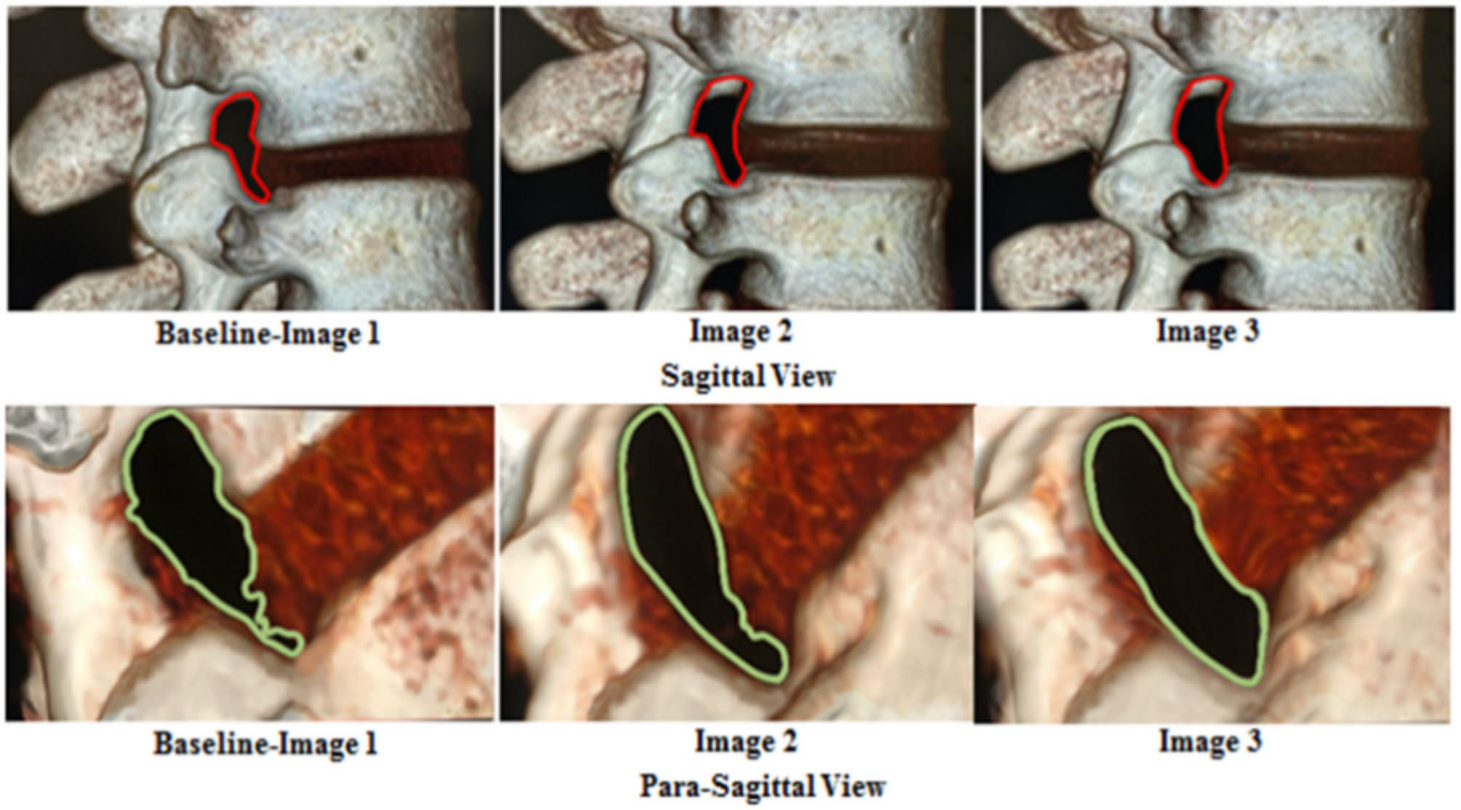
3D-CT scan images of the CSA of the LIVF at L5/S1 level
